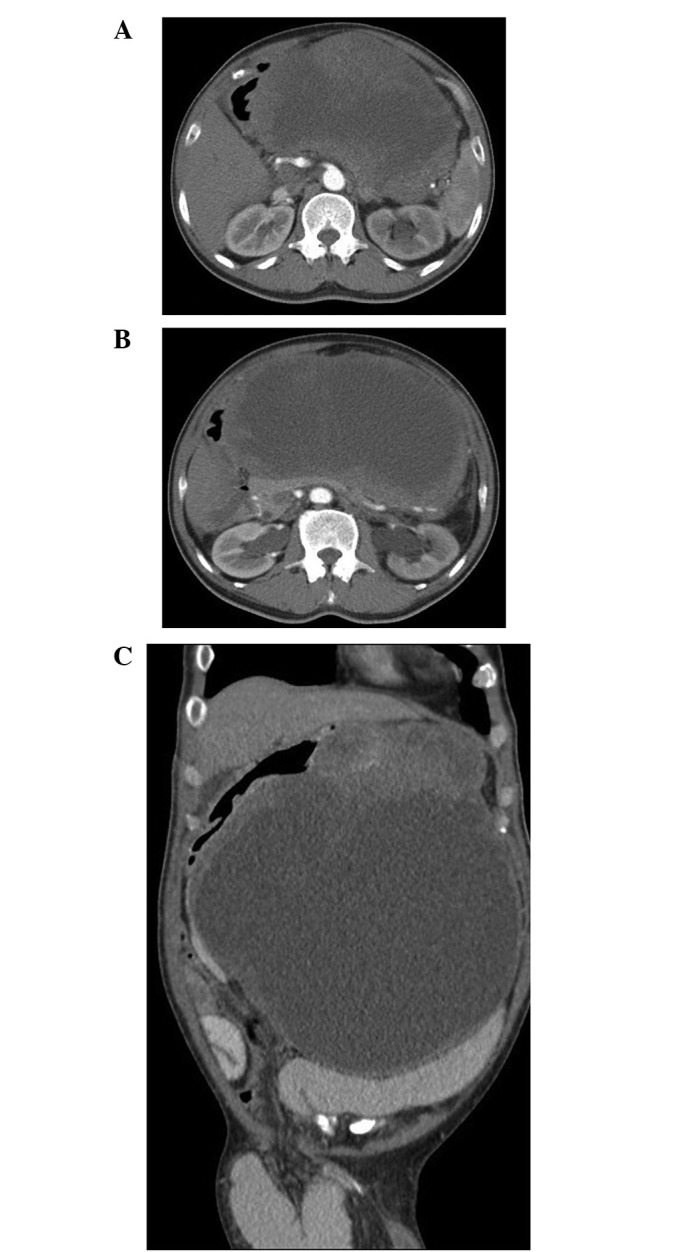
Computed tomography scan shows a large cystic-based tumor with a wall of uneven thickness. The tumor, with an unclear boundary between the stomach and spleen, (A) compressed the stomach to the right upper quadrant, leading to gastric lumen narrowing, (B) extended backward and compressed the pancreas and (C) almost occupied the entire abdominal cavity.
